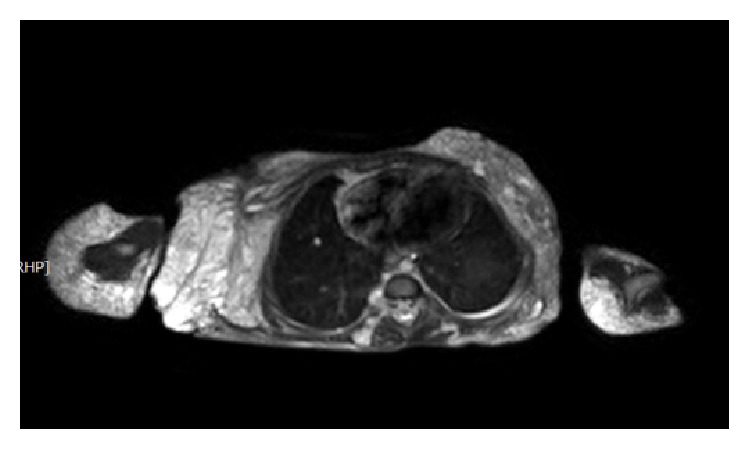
MRI of the chest (phase T2 sequence, with fat suppression), showed the lymphangioma in the upper part of the chest wall, in the right arm.
